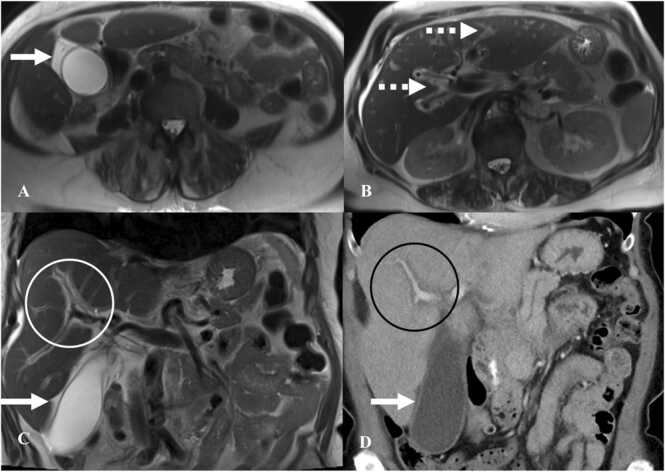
Immune-mediated hepatitis in a 68-year-old man with melanoma on Pembrolizumab. A&B. Axial T2W images of MRI of the abdomen show gallbladder wall edema (white arrow) and periportal edema (dashed white arrow). C. Coronal T2W MRI abdomen image shows periportal edema (white circle) and gallbladder wall edema (arrow). D. Coronal image on contrast-enhanced CT of the abdomen shows periportal edema (black circle) with gallbladder wall edema (arrow). Also note diffuse hypoattenuation of the liver.
